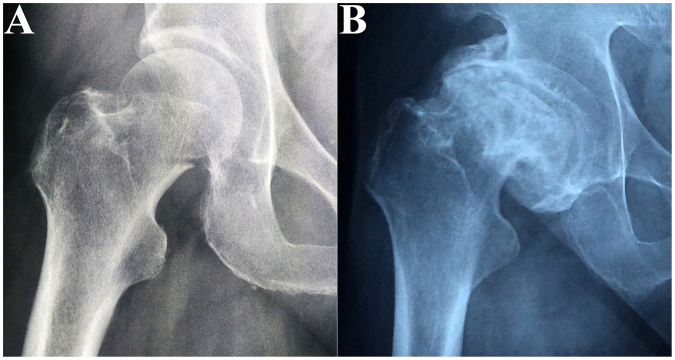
Anteroposterior X-ray of included patients. (A) Femoral neck fracture; (B) Femoral head necrosis.
